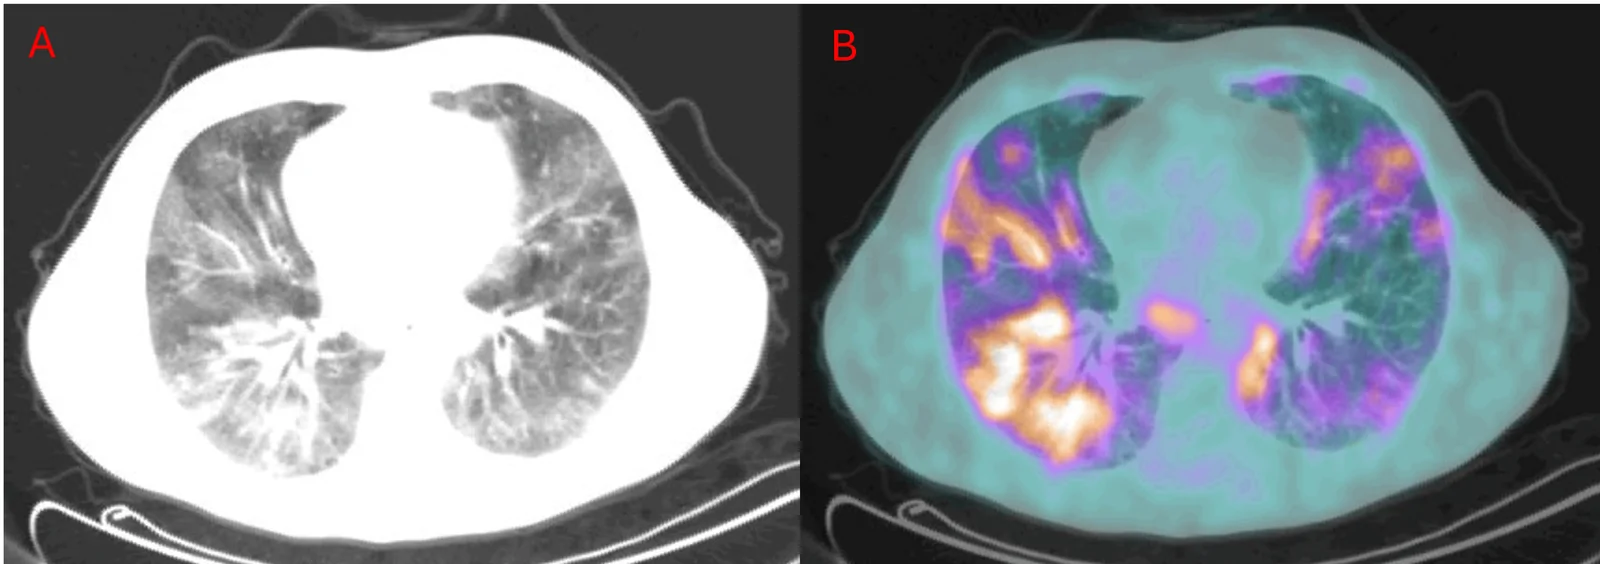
Thoracic findings on 18F-fluorodeoxyglucose PET/CT. (A) Axial CT image showing bilateral pulmonary ground-glass opacities. (B) Fused axial ^18^F-FDG PET/CT image demonstrating increased metabolic activity in the pulmonary parenchyma and hilar regions.
